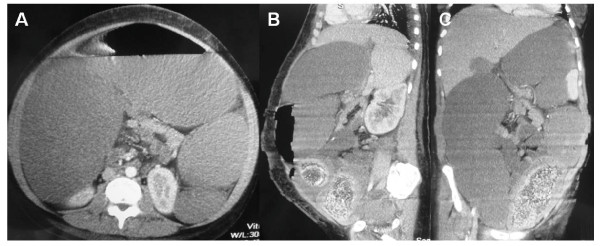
A, B and C: Axial, sagittal and coronal abdominal CT scan images showing dilated colon.
